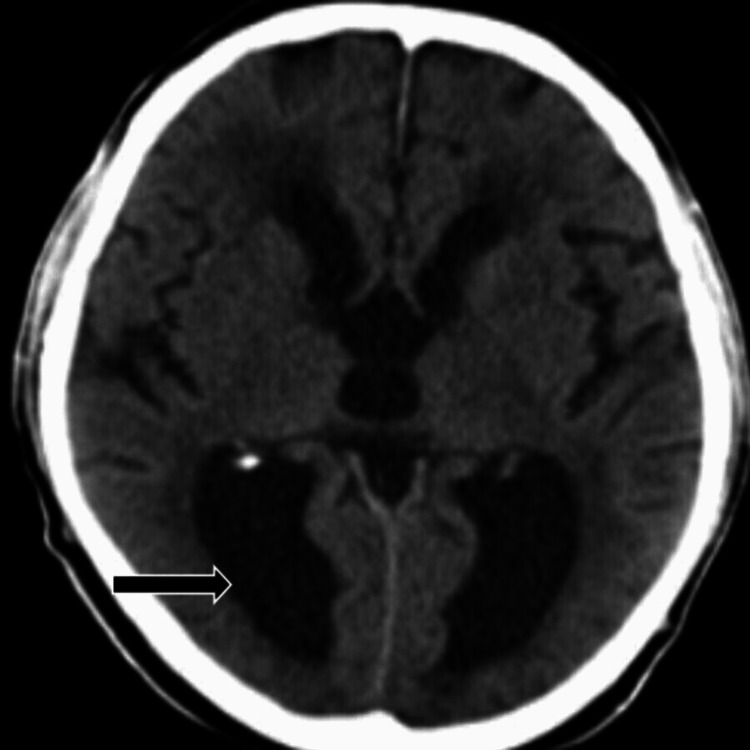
CT of the brain showing communicating hydrocephalus with periventricular ooze
